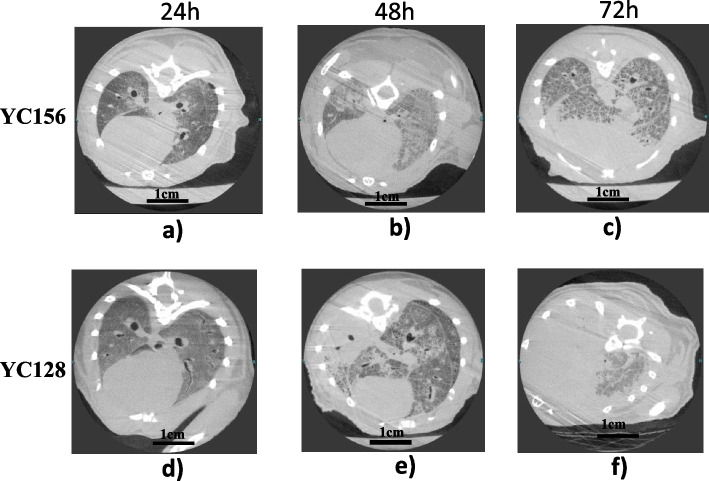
YC128 induced more severe imaging changes shown in chest CT scan. a 24 h after YC156 inoculation. Slight patchy opacities in both lungs. b 48 h after YC156 inoculation. Moderate patchy opacities in both lungs. c 72 h after YC156 inoculation. Patchy opacities alleviation in both lungs. d 24 h after YC128 inoculation. Slight patchy opacities in both lungs. e 48 h after YC128 inoculation. Complete consolidation was present in the whole left lung and local patchy opacities in the right lung. f 72 h after YC128 inoculation. consolidation appeared in bilateral lungs like “white lung”
